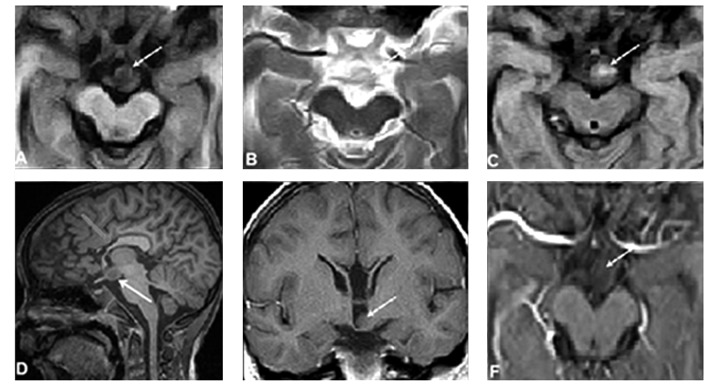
Pre-surgical 3.0 T magnetic resonance imaging (MRI) examination of the patient. (A-E) MRI revealed the ovoid-shaped hypothalamic hamartoma (HH) located at the the suprasellar region (indicated by arrow). (F) No enhancement of the HH MRI with intravenous, gadolinium-based contrast (indicated by arrow). (A) T1-weighted spin-echo (SE) axial slice. (B) T2-weighted SE axial slice. (C) Fluid attenuated inversion recovery (FLAIR) axial slice. (D) T1-weighted SE sagittal slice. The corpus callosum with absence of the rostrum, the genu and frontal part of the body of corpus callosum (indicated by arrow). (E) T1-weighted SE coronal sclices. (F) T1-weighted SE axial enhanced slice.
